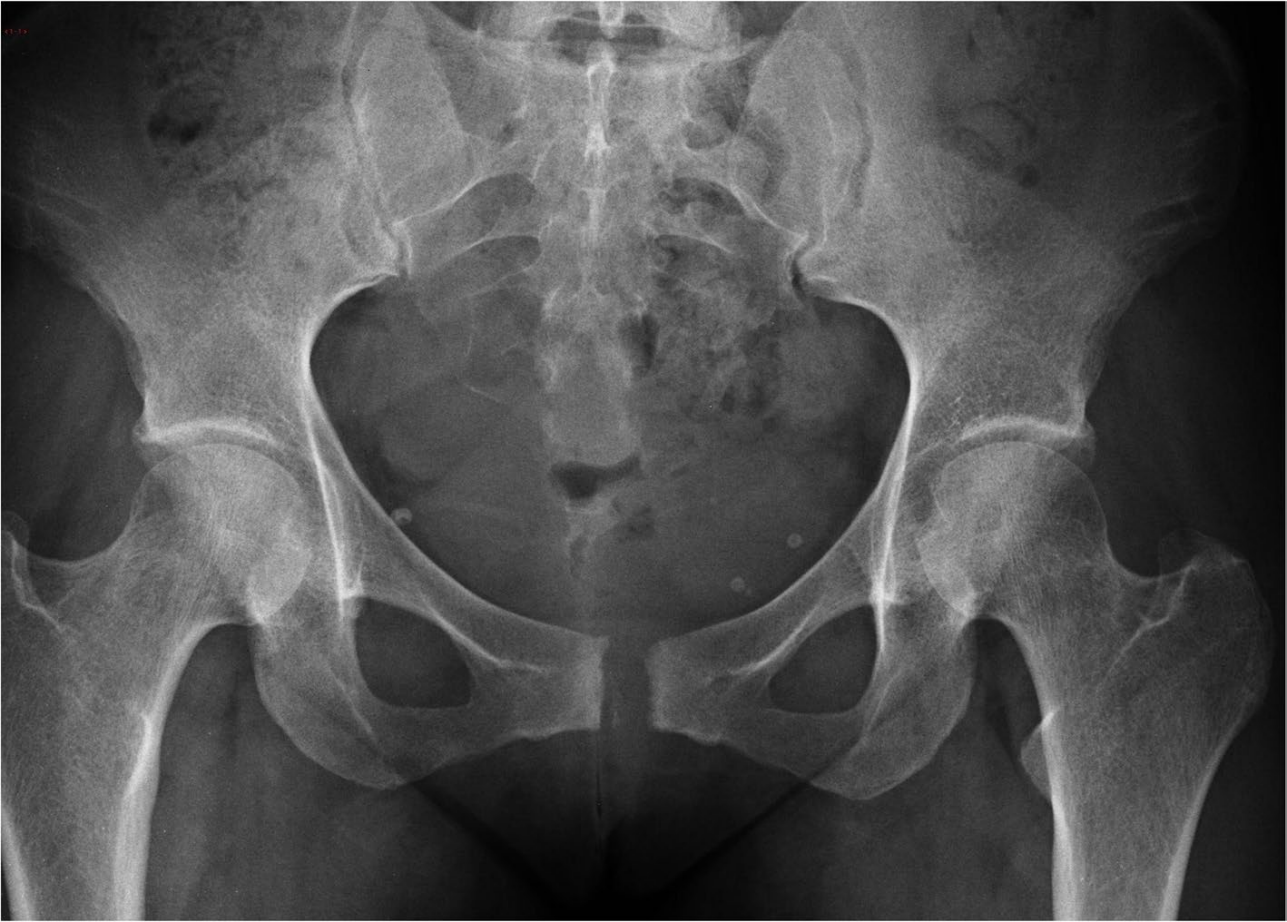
Case 1. A 34-year-old woman was treated with an external fixator with excellent results. A radiograph taken 1 year postoperatively showed a 12 mm diastasis
